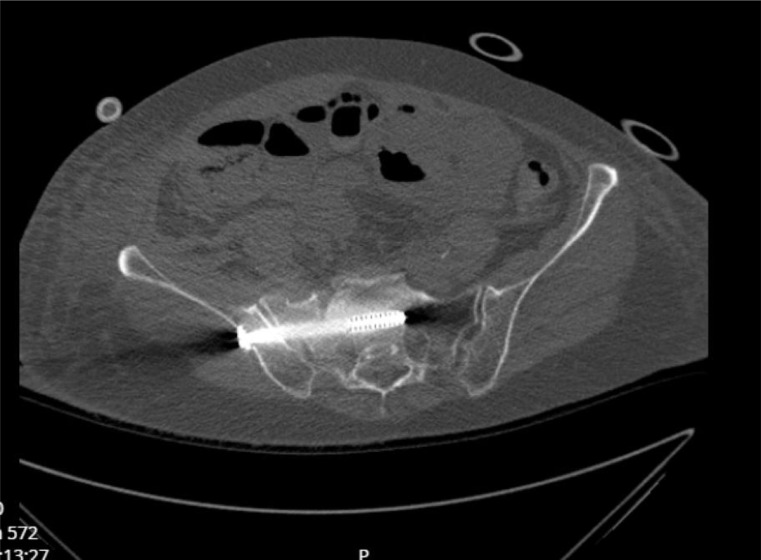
Example of a correctly placed SI screw. Axial view of CT scan. The SI screw is positioned in the cancellous bone, without traversing the neural foramen or anterior cortical protrusion
